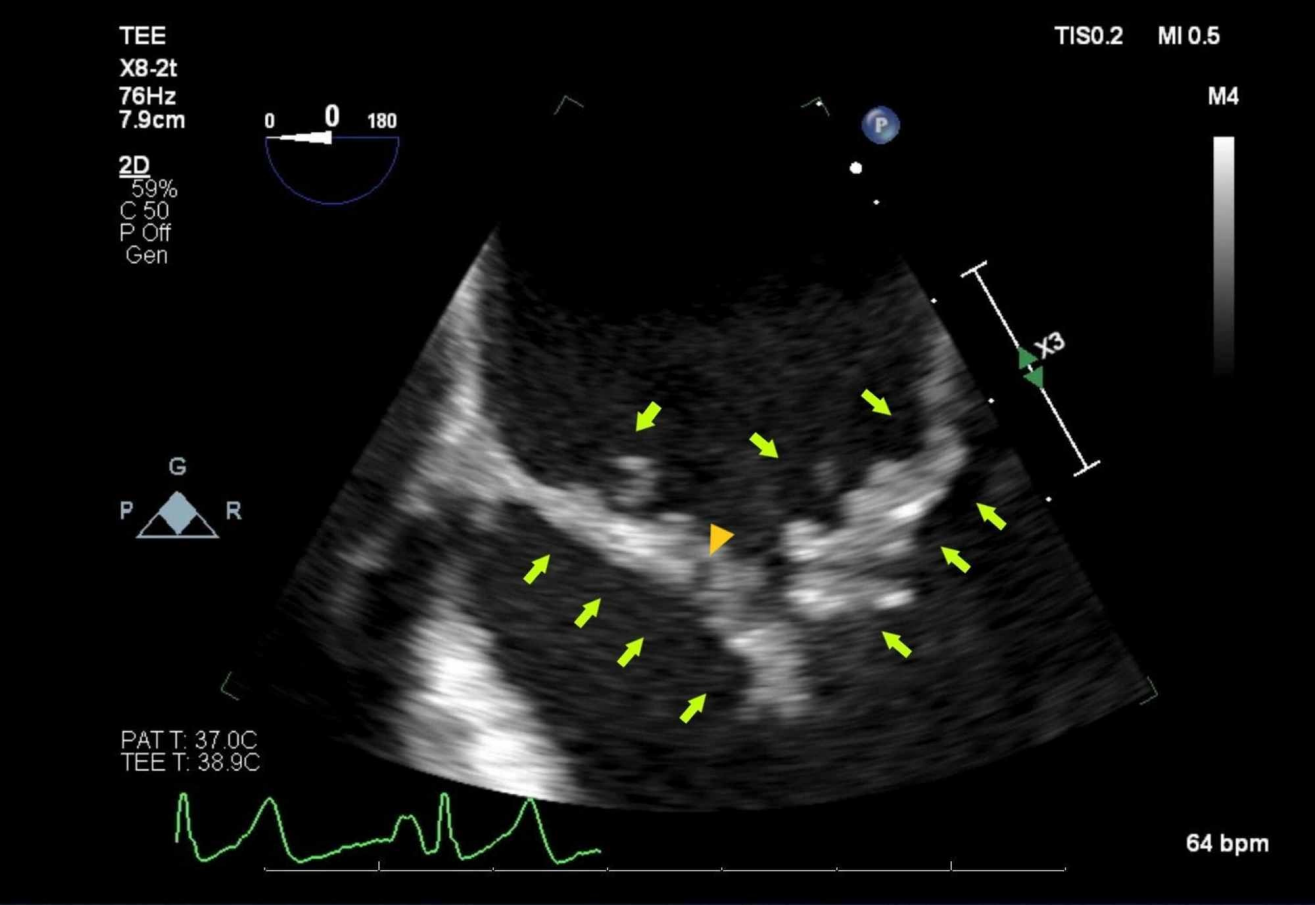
Transesophageal echocardiogram (TEE) revealing multiple vegetations along the mitral valve (arrow) and a central area of lucency consistent with an abscess (arrowhead)
